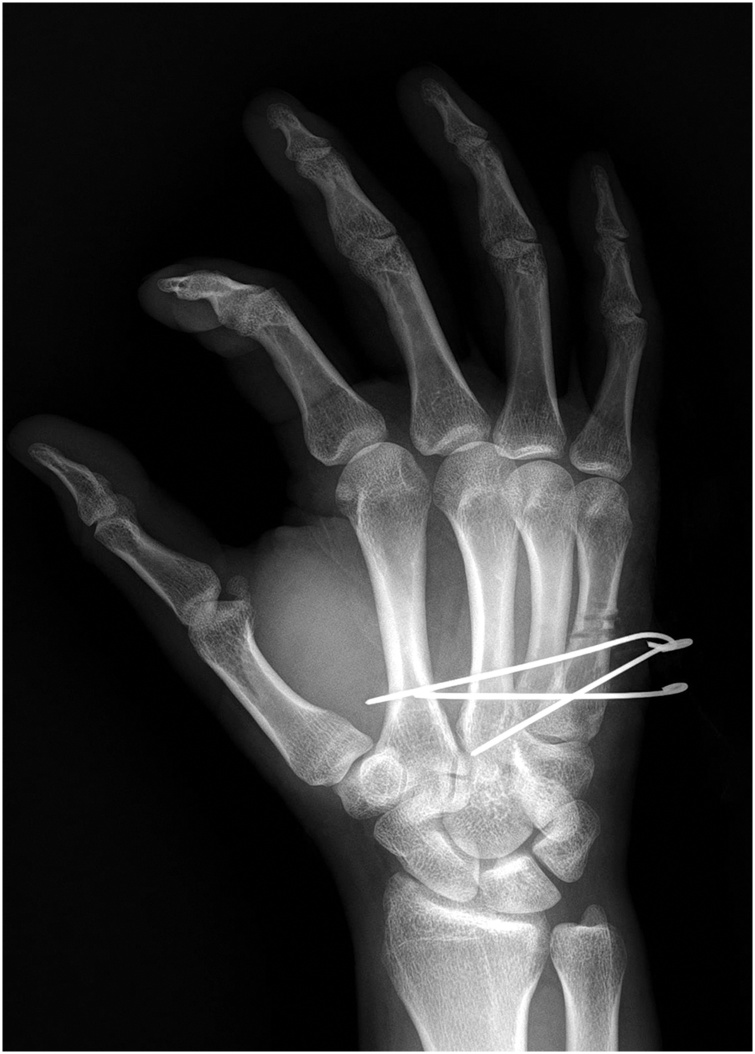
Simple X-ray at five weeks postoperatively. Simple X-ray of his hand demonstrated the union of the fractured site but the K-wire track still showed with no signs of displacement.
